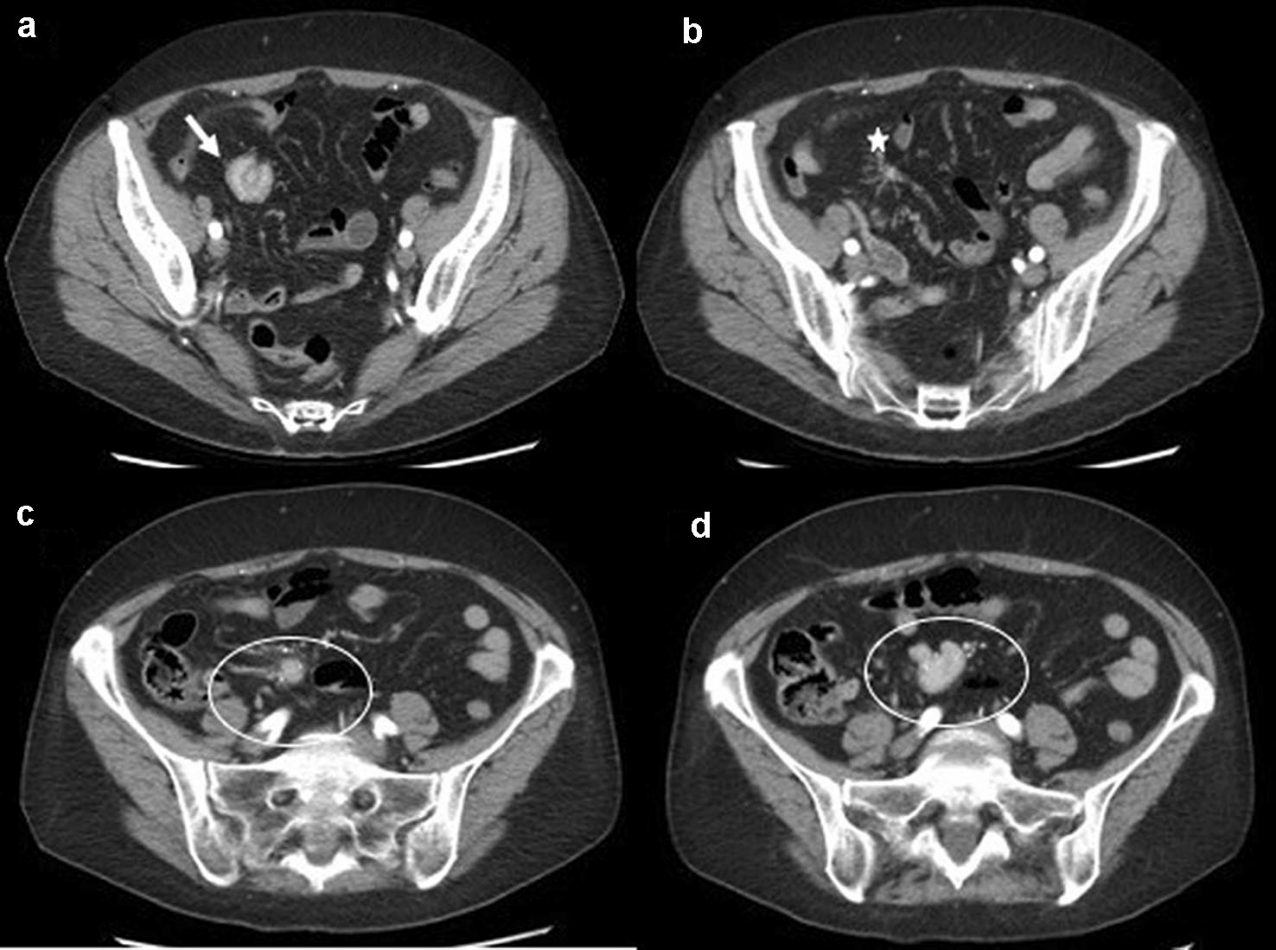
Gastrointestinal neuroendocrine neoplasm (G3) in the terminal ileum. Axial contrast-enhanced CT image in the arterial phase (a) demonstrates a bowel wall thickening (white line) of the terminal ileum and (b) a stellate soft tissue nodule (white star) in the mesentery. Under, there are many lymph nodes metastases (white circle) (c, d)
